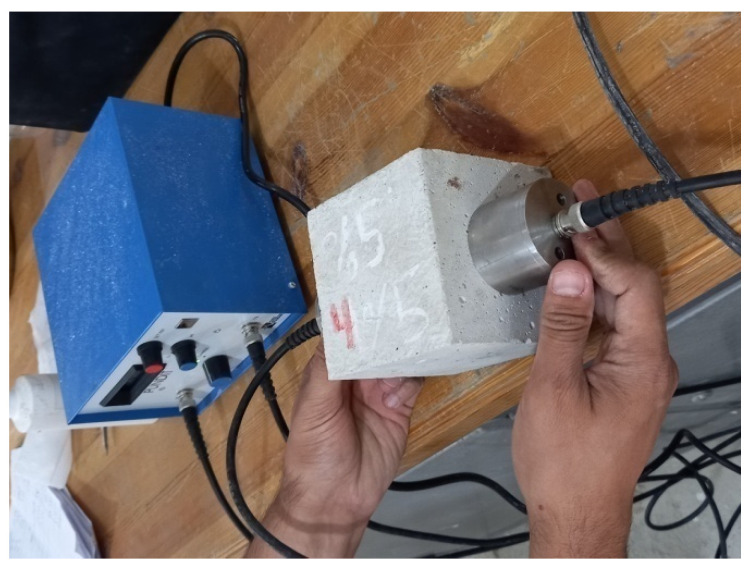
Ultrasonic pulse velocity test setup.
